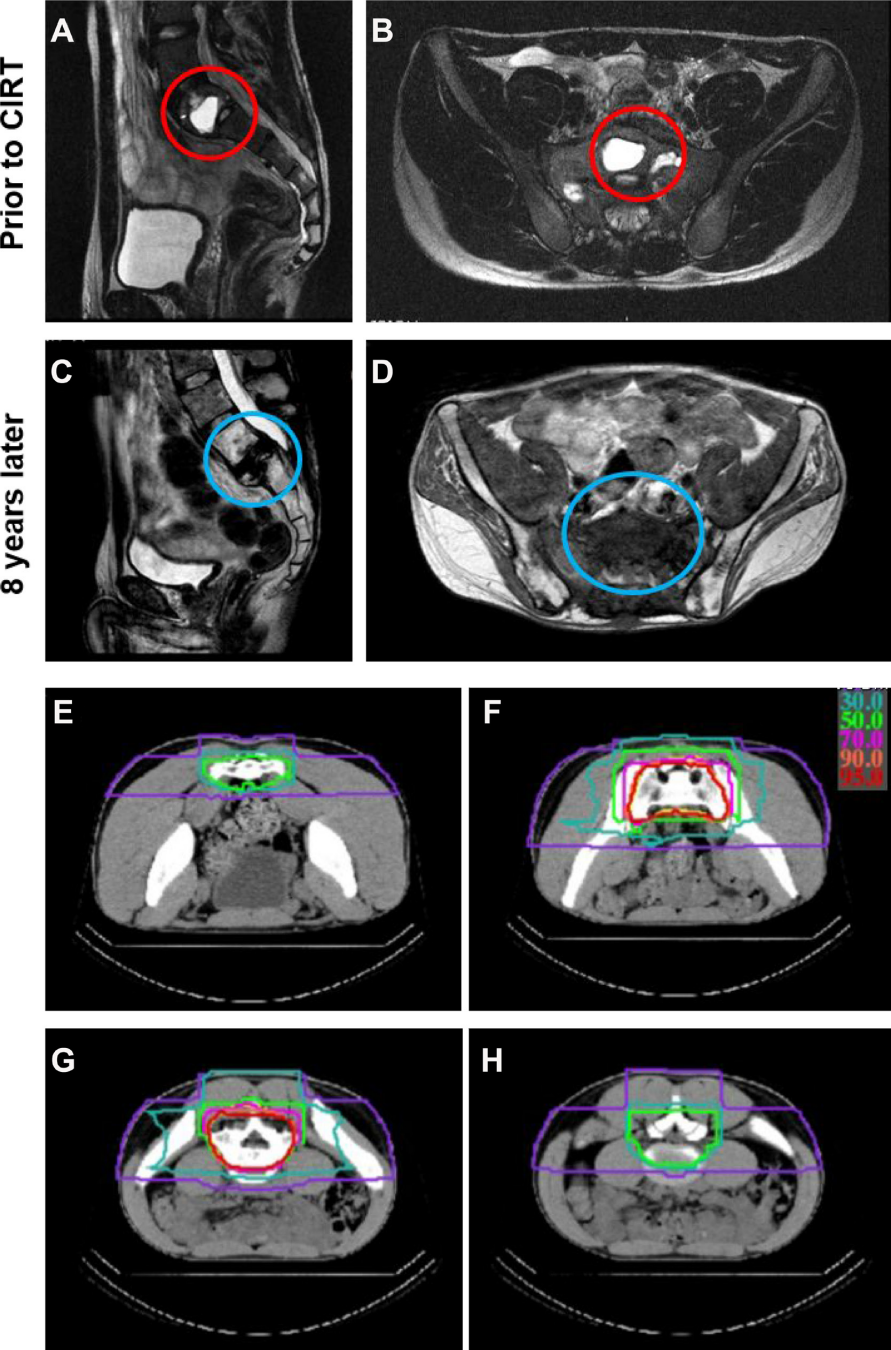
An example of a treated case with unresectable sacral (S1) osteosarcoma (A–B) show magnetic resonance imaging (MRI) scans prior to carbon ion radiotherapy (CIRT) in the sagittal (A) and horizontal (B) planes with the tumor highlighted in the red circles. (C–D) show MRI scans 8 years after CIRT in the sagittal (C) and horizontal (D) planes without any tumor recurrence (blue circles) but with obvious fracture in S1. (E–H) show selected computed tomography (CT) cross sectional views of the CIRT dose distribution (isodose lines: red 95%, orange 90%, pink 70%, light green 50%, green 30%, purple 10%).
